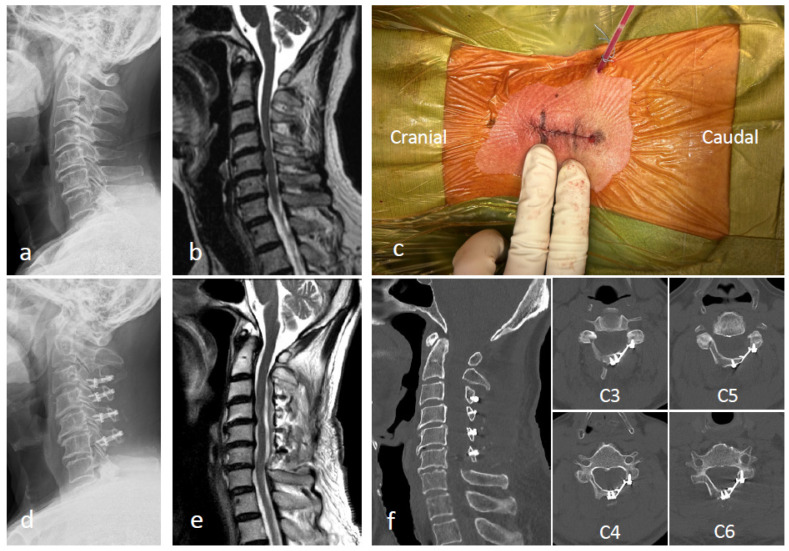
(a,b) Preoperative radiography and magnetic resonance imaging (MRI) images. (c) Exoscopic minimally invasive open-door laminoplasty (exLAP) is performed through a small incision with a length of two fingers. (d–f) Postoperative radiography, MRI, and computed tomography images after exLAP at C3–C6.
